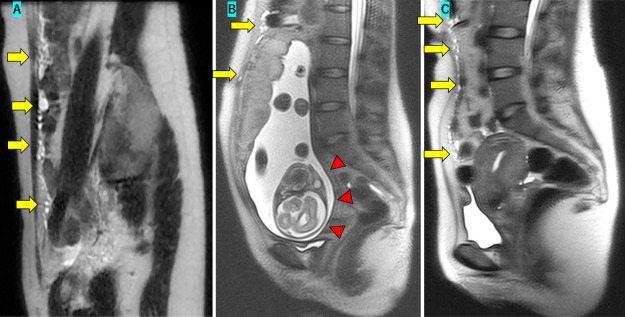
A MRI image on presentation showing multiple cysts (arrows) in the omentum, B MRI image obtained at 28 weeks’ gestation showing multiple cysts (arrows) and a fetus (triangle), C MRI image obtained one month after delivery showing multiple cysts (arrow), as in previous MRIs
